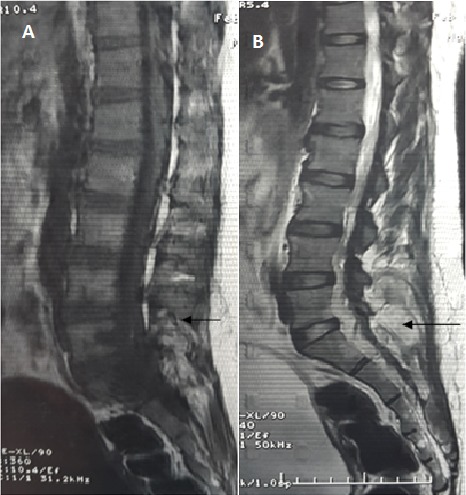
Preoperative MRI shows lesion with low signal in T1WI and heterogeneous high signal in T2WI
